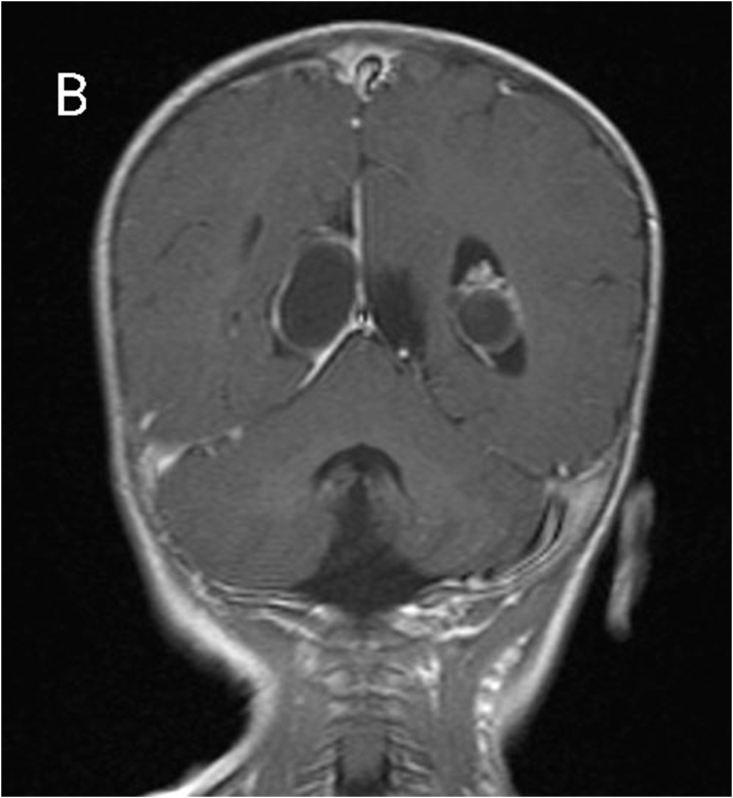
coronal T1-weighted image showing multiple cysts with peripheral enhancing.
